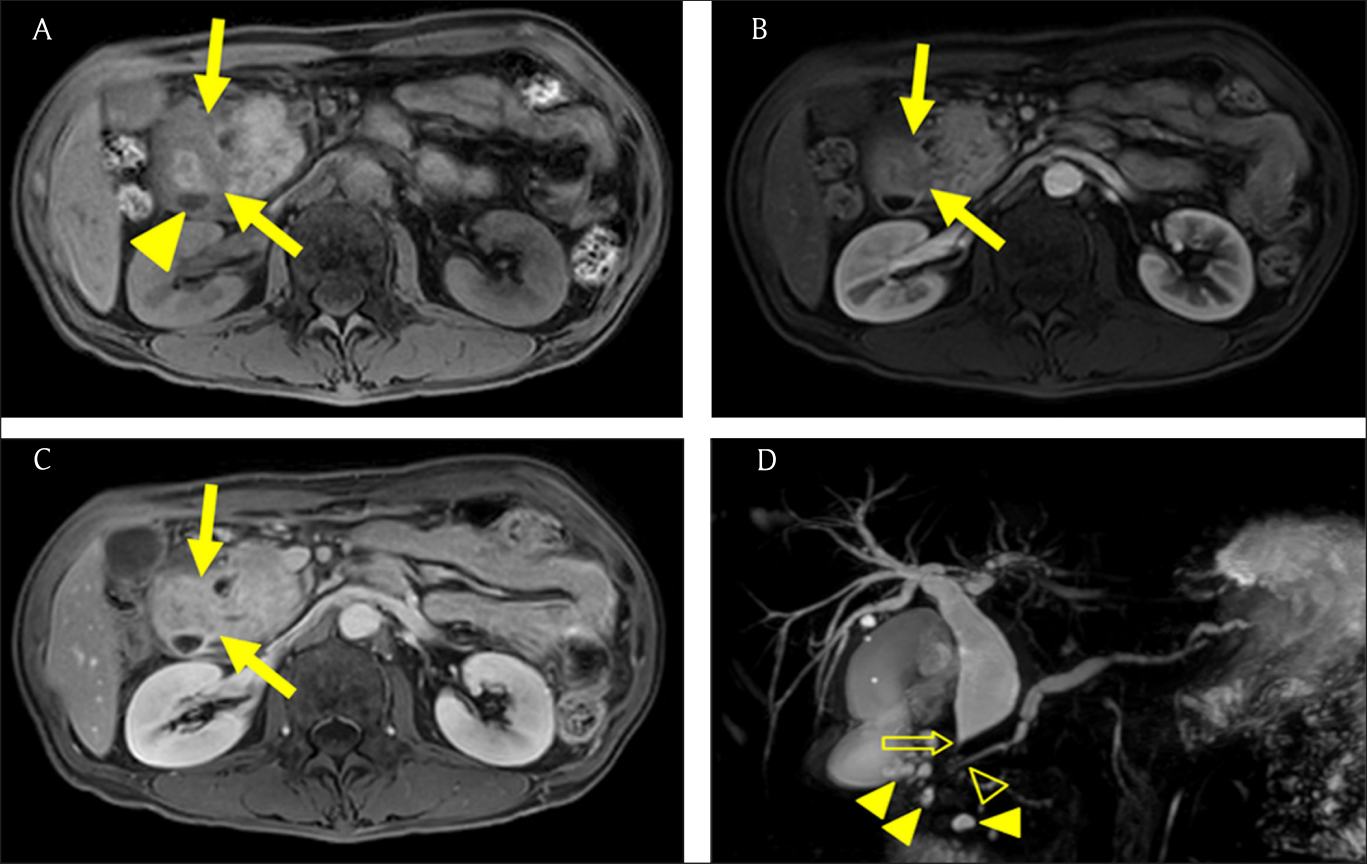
A 49-year-old man with groove pancreatitis who had a history of alcohol consumption greater than 50 g/day for more than 20 years. (A) Axial T1-weighted image shows a hypointense lesion (arrows) in the pancreaticoduodenal groove. There is an oval low signal intensity lesion (arrowhead) in the duodenal wall, suggesting a cyst. (B, C) Axial gadolinium-enhanced arterial and 3-minute delayed phase MR images show delayed enhancement of the mass-like lesion (arrows). (D) MRCP image shows dilatation of the bile and pancreatic ducts with smooth tapered narrowing (open arrow and open arrowhead). Note multiple cystic lesions (arrowheads) around the pancreaticoduodenal groove.
